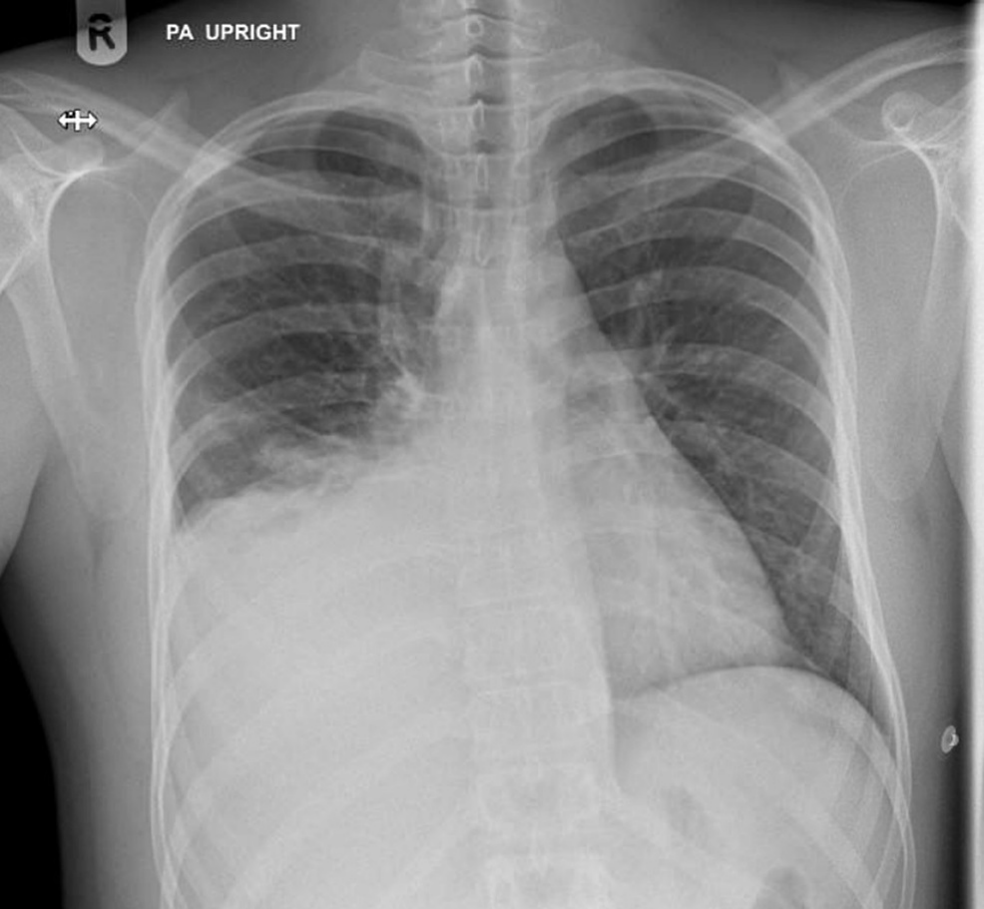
Chest X-ray shows right-sided pleural effusion with the possibility of mass lesion along the right lower hemithorax.
